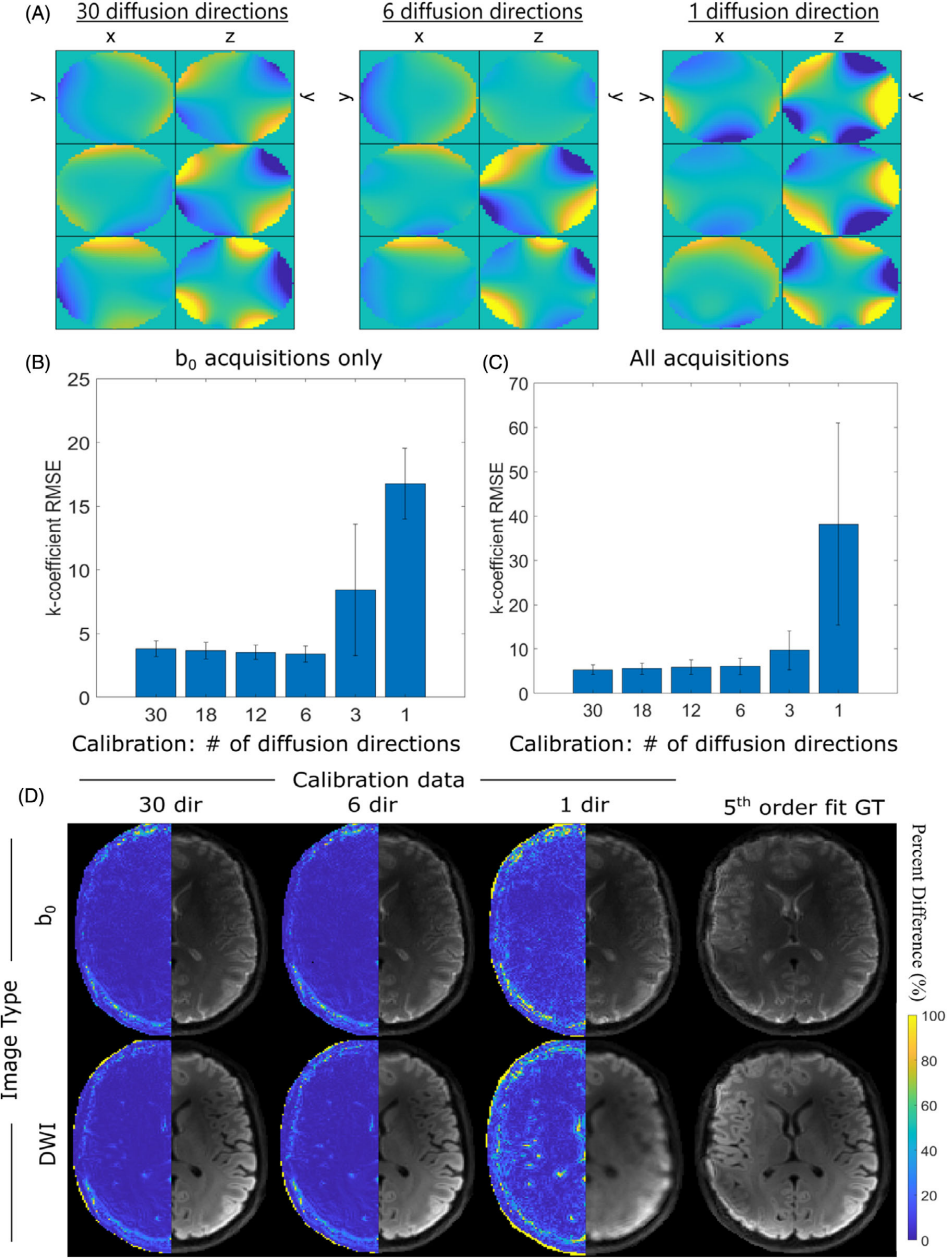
(A) Cross‐sectional spatial distribution (left‐to‐right: x‐y, y‐z planes) of compressed basis functions for calibration data equipped only with diffusion data containing 30, 6, 1 directions. b_0_ Acquisitions were omitted from the calibration data itself for all relevant analysis. Rows represent the new basis functions related to the first three principal components. Mean root‐mean‐square‐error (RMSE) analysis of up‐to‐second‐order k‐coefficients, when preserving the following amount of uniform diffusion directions in calibration: 30, 18, 12, 6, 3, 1, for (B) b_0_ acquisitions only, and (C) when including the diffusion acquisitions in the analysis. (D) Mean b_0_ (top) and DWI (bottom) reconstructions when informed by field dynamics compressed using (left‐to‐right) 30, 6, 1 diffusion directions in the calibration data, plus respective fifth order fits calculated using 100 probes. Percent difference images were calculated relative to images informed by fifth order field dynamics and are shown in the left hemisphere of the images. Five singular values were kept for compression. Directions in the subsets were chosen to maximize electrostatic repulsion for each subset.
